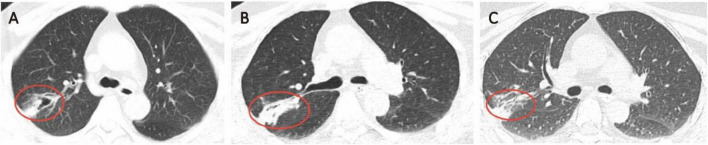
(A) Chest CT at admission. (B,C) Follow-up Chest CT.
